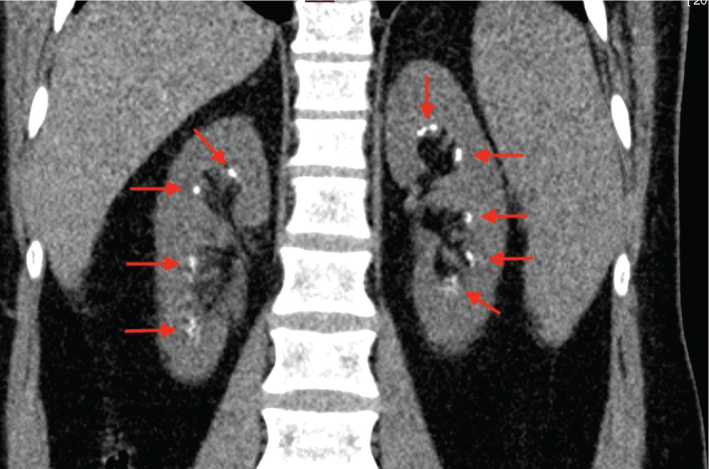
Bilateral nephrocalcinosis. Bilateral nephrocalcinosis in computed tomography (red arrows).
